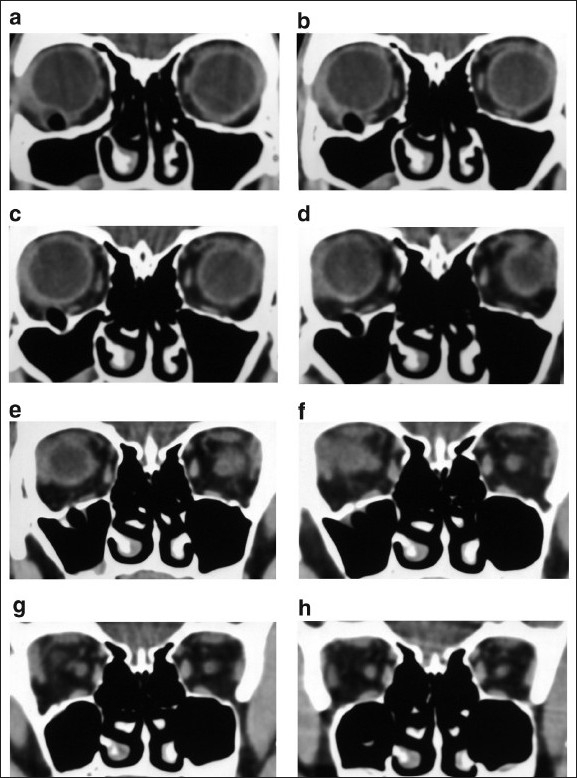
(a-h) Sequential coronal computed tomography scan of patient showing well-defined radiolucent foreign body passing posteriorly and caudally in maxillary sinus with orbital floor fracture
